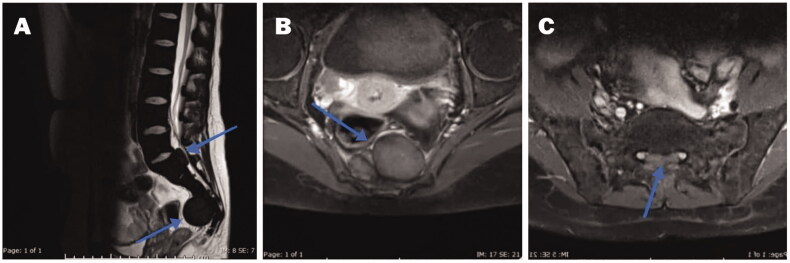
MRI spine: (A) Sagittal T2 WI showing a diffuse low-signal intensity of the bone marrow of a well-defined isointense mass (5.3 × 3 cm) in the presacral region (blue arrow). A second mass (2 × 1 cm) in anterior extradural space posterior to S1 level (Blue arrow). (B) Mass enhancement seen in the axial postcontrast fat-saturated image at the presacral area (Blue arrow). (C) Axial postcontrast fat-saturated image at S1 level showing anterior extradural mass with slightly high signal intensity (blue arrow) at the midline, with displacement of the S1 nerve roots laterally. *Note*. Image taken from Clinical Case reports from a previously published article [42]. The licence of the article (CC-BY) permits unrestricted reuse of the published work.
